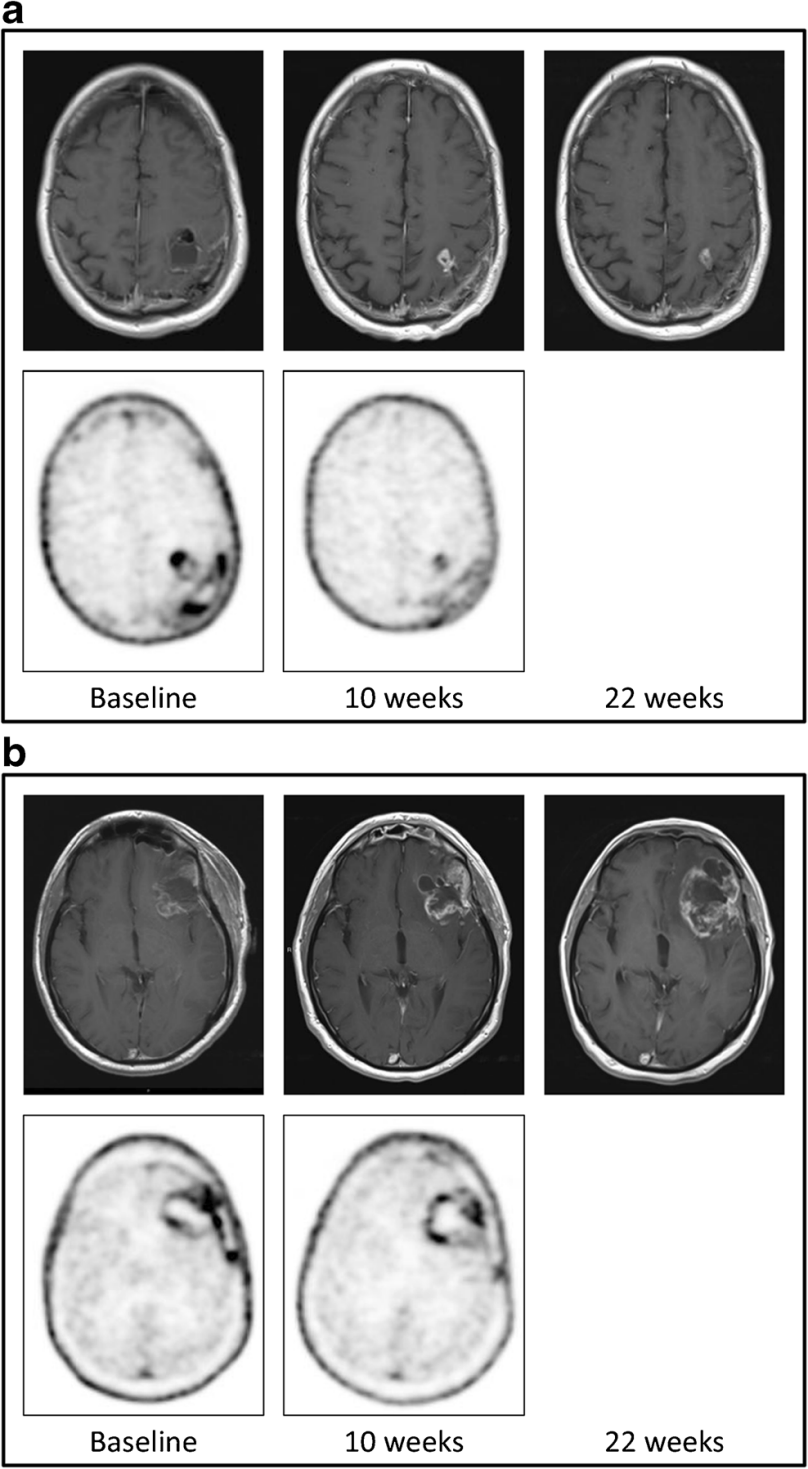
MRI and FLT PET imaging in a patient with (a) pseudoprogression and (b) true progression: *top row*: MRI images at baseline (*left*), 10 weeks (*centre*) and 22 weeks (*right*); *bottom row*: PET images at baseline (*left*) and 10 weeks (*right*). FLT PET images showed a SUV_max_ of 1.44 at baseline and 0.74 at 10 weeks in the patient with pseudoprogression, and 3.70 at baseline and 1.80 at 10 weeks in the patient with true progression
